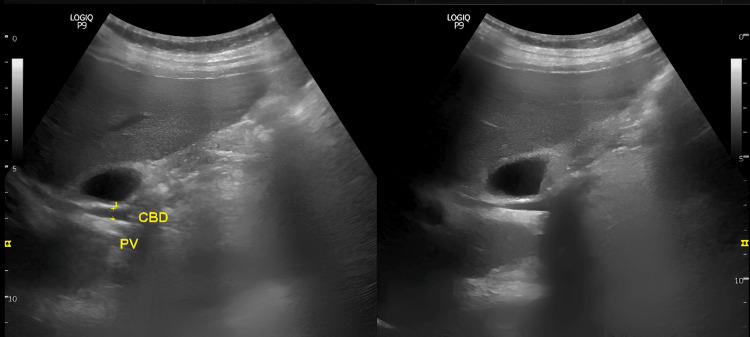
Ultrasonographic measurement of the proximal common bile duct (CBD) Representative longitudinal sonographic image obtained at the porta hepatis using a 3.5 MHz curvilinear transducer. The CBD (arrow) is visualized anterior to the portal vein and measured at the proximal level.
